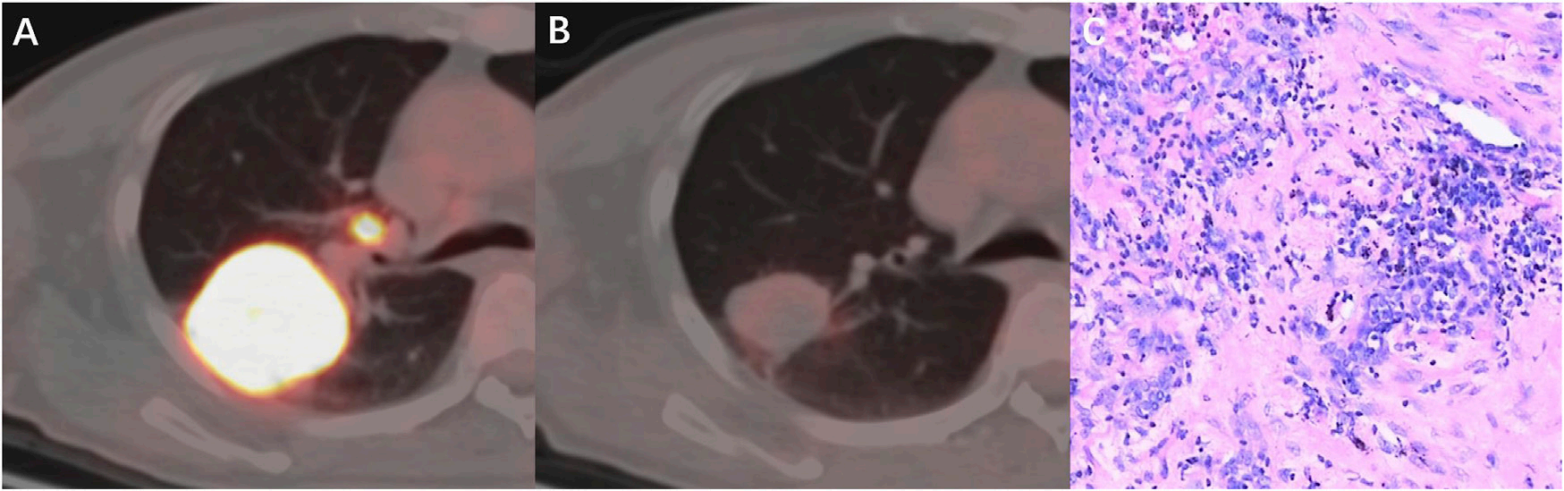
A 53-year-old man with squamous cell carcinoma, who had marked metabolic changes on PET scanning after two cycles of neoadjuvant immunochemotherapy and was classified as responder per iPERCIST-max. (A) Baseline PET-CT fusion image of the primary tumor and lymph nodes; (B) Follow-up PET-CT fusion image showed markedly reduced metabolic activity of the tumor. (C) pathological examination revealed this patient had mPR disease. mPR, major pathological response.
